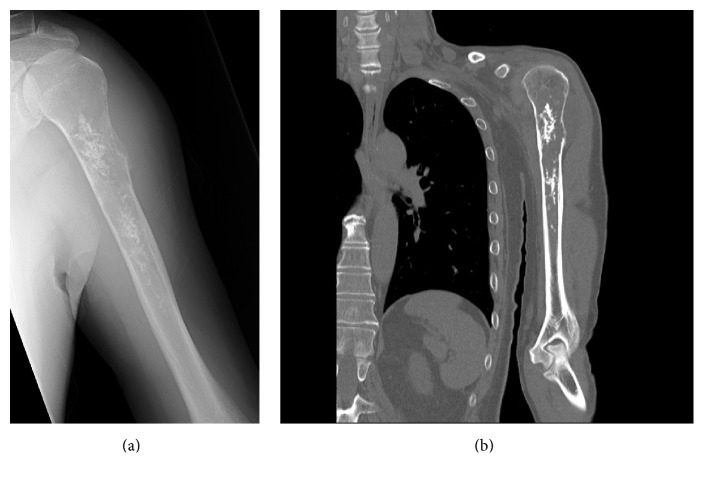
(a) A plain radiography and (b) CT of the left humerus. Bone marrow calcification and cortical expansion of the proximal humerus are observed.
